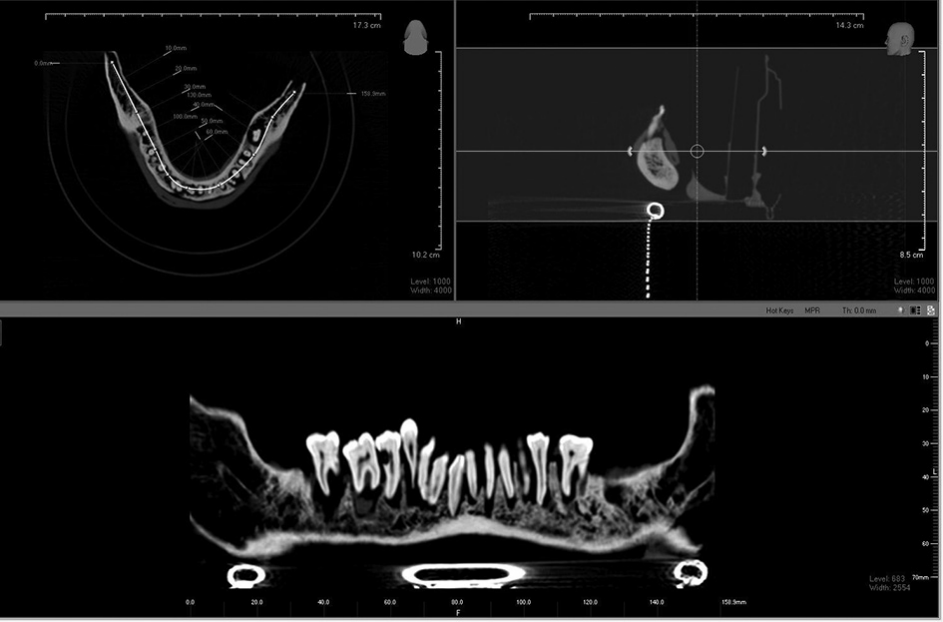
Panoramic reconstruction of CBCT images
